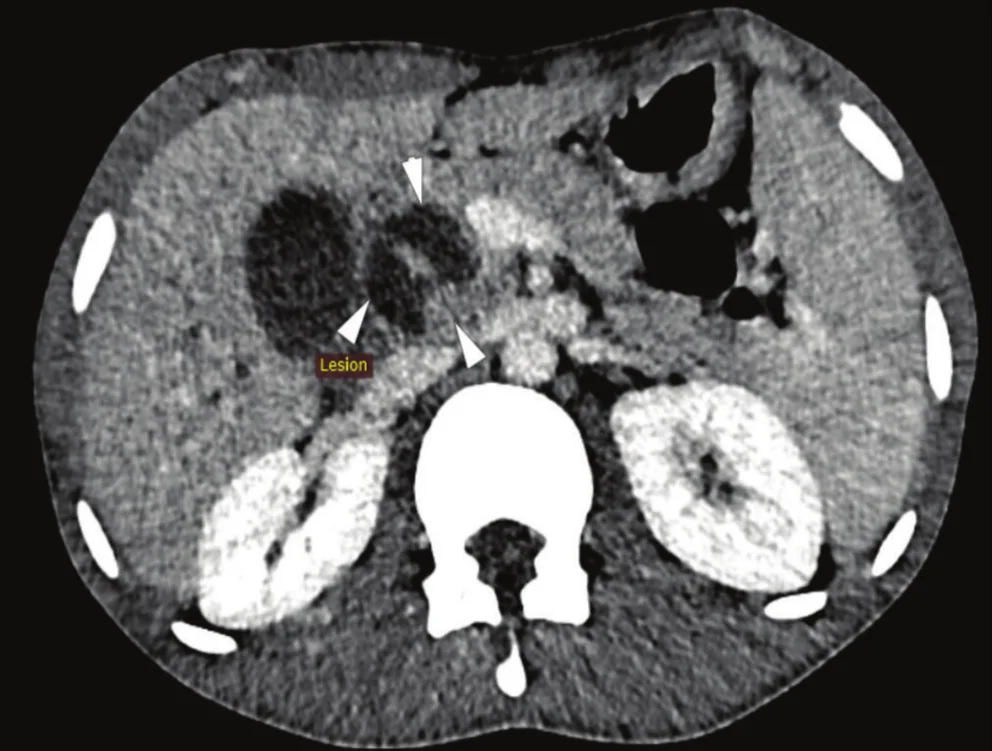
Axial contrast‐enhanced computed tomography image at the level of the porta hepatis showing a poorly circumscribed, heterogeneously attenuating periportal mass lesion measuring 3.3 × 3.5 cm, with heterogeneous contrast enhancement and a prominent non‐enhancing component.
